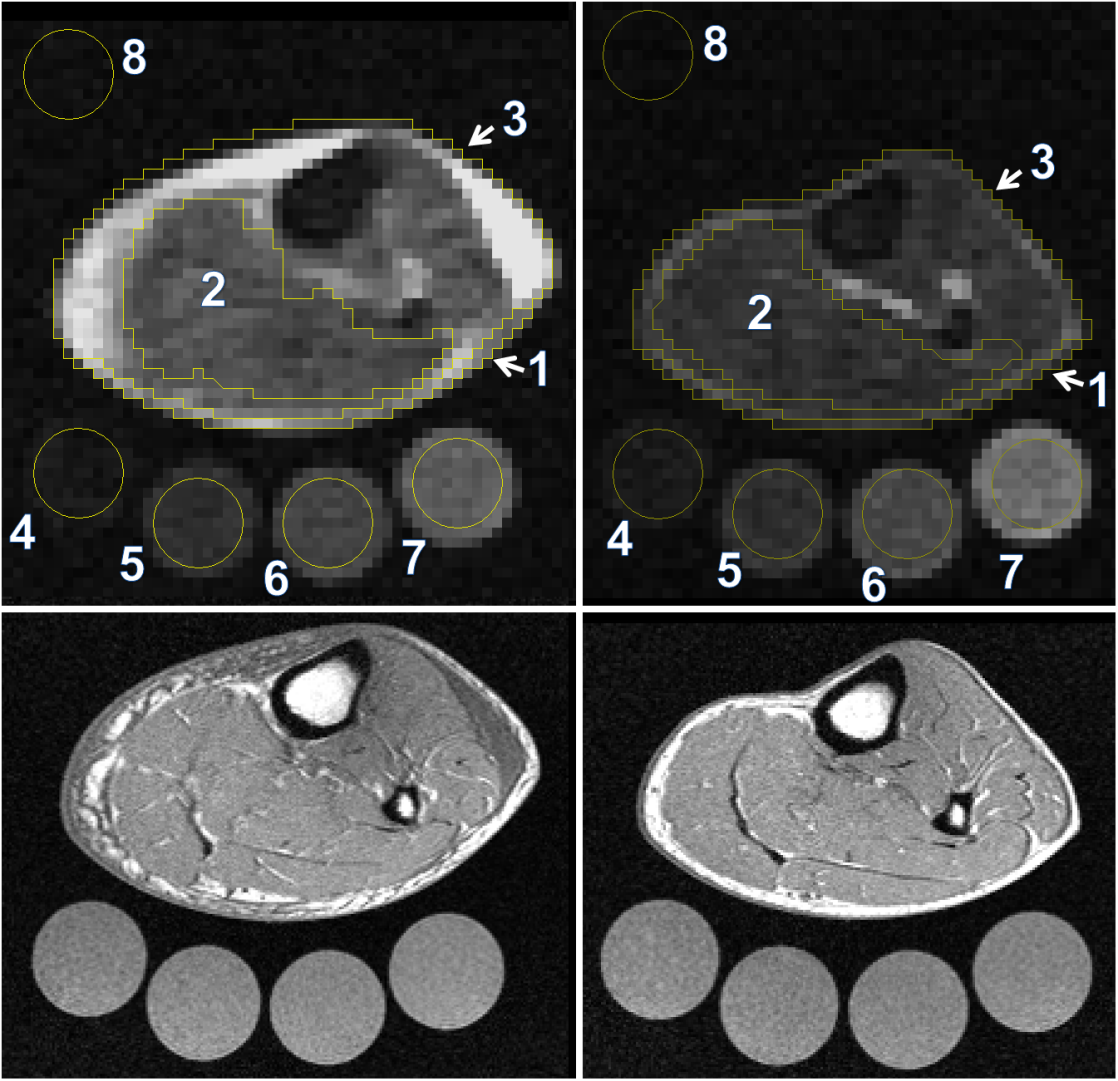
Evaluation of the compartments of the lower leg. The compartments of the lower leg were determined before (left) and after (right) diuretic therapy by an experienced reader. The different anatomical regions of interest (ROI) were drawn guided by the anatomical image (lower images, T1-weighted fast-low-angle-shot (FLASH)-sequence). To measure the cutis one pixel was marked along the coil surface. The value of the whole lower leg = grey-values > 2 x background noise. Four tubes containing aqueous solutions with increasing Na^+^ concentrations (10, 20, 30, and 40 mmol/L NaCl) were positioned in a custom-made device positioned inside the coil just below the patient’s lower leg. Gray-scale measurements of the tubes served as calibration standards for ^23^Na-MRI of the skeletal muscle and skin by relating intensity to a concentration in a linear trend analysis. For H_2_O quantification, the 10 mmol/L NaCl tube served as a calibration standard for tissue water in a linear trend analysis defining a water content of approximately 1 liter water per liter volume. ROIs: 1 = cutis, 2 = triceps surae muscle, 3 = whole lower leg, 4–7 = calibration solutions, 8 = background noise.
